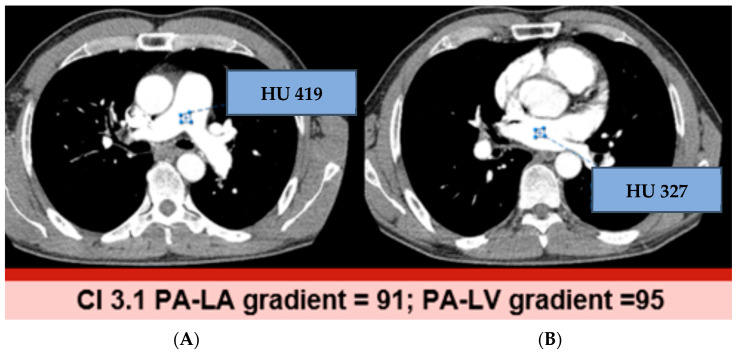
CTEPH case with normal cardiac index. Post contrast CT axial image at the level of MPA with ROI in the MPA (A) and LA (B). The MPA-LA gradient was 91, which is below the cut-off of 102 that correlates with normal cardiac index (≤102 implies normal CI).
